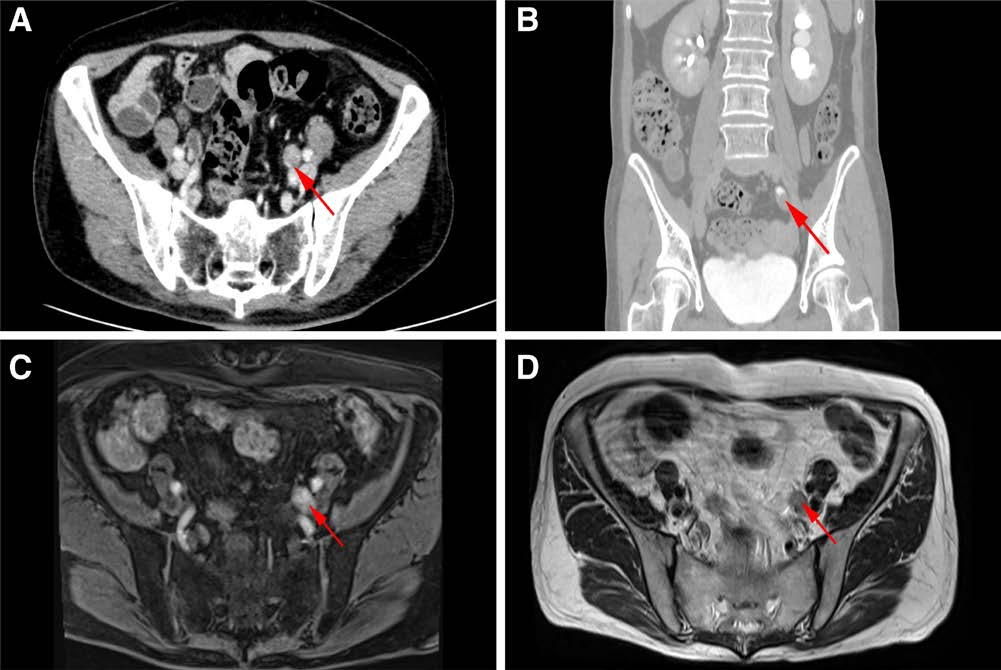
CT urography enhancement phase (A) shows a long-striated enhancing lesion in the left mid-ureter and dilatation of the ureter and renal pelvis above the lesion combined with hydronephrosis; CT urography excretion phase coronal (B). Pelvic MRI: T1WI (C) showed an isotropic T1 markedly intensified signal shadow in the middle part of the left ureter, with a size of about 1.5 × 1.1 × 2.1 cm; T2WI (D) showed a slightly longer T2 signal shadow in the lesion area. The red arrow points to the lesion. CT = computed tomography, MRI = magnetic resonance imaging.
